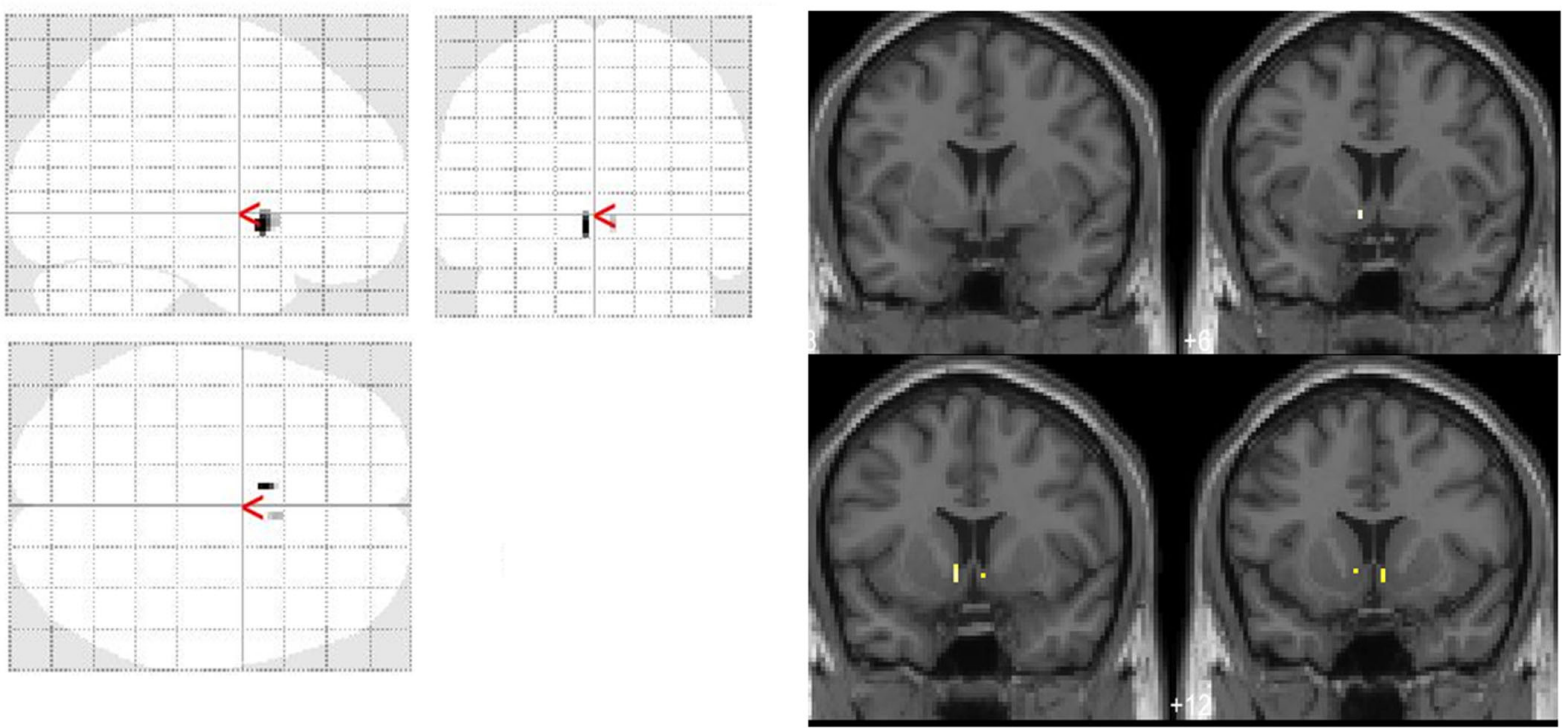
The left figure shows the Statistical Parametric Mapping (SPM) in the standard format as a maximum intensity projection viewed from the right, back, and top of the brain. Two significant clusters (*p* < 0.05, uncorrected) were observed in the left nucleus accumbens (peak Montreal Neurological Institute [MNI] coordinates: −6, 8, and −6) and right nucleus accumbens (peak MNI coordinates: 6, 12, and −6). The right figure shows significant clusters on coronal magnetic resonance imaging (MRI), showing a significant reduction in dopamine transporter (DAT) availability in the bilateral nucleus accumbens.
